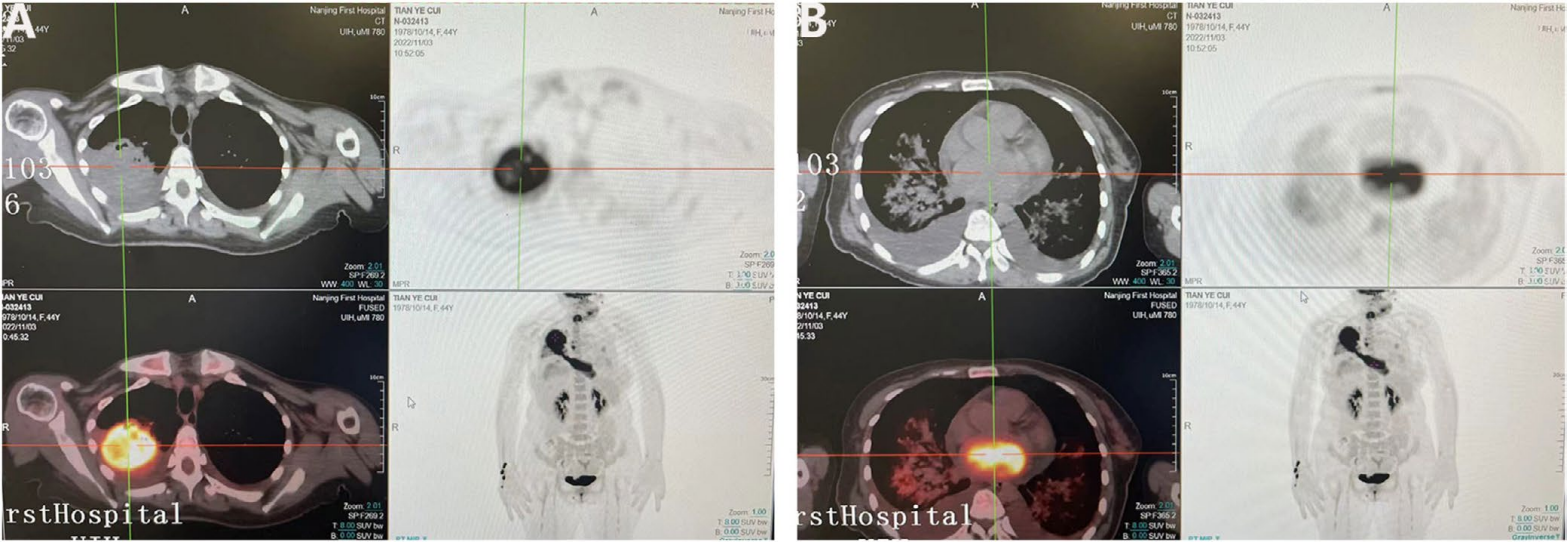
PET‐CT. (A) FDG‐avid mass in the right upper lobe measuring 72 mm × 68 mm, suggestive of a primary malignant lung tumor. (B) FDG‐avid mass in the left atrium extending along the right upper pulmonary vein and continuous with the pulmonary mass, suggestive of a tumor thrombus.
